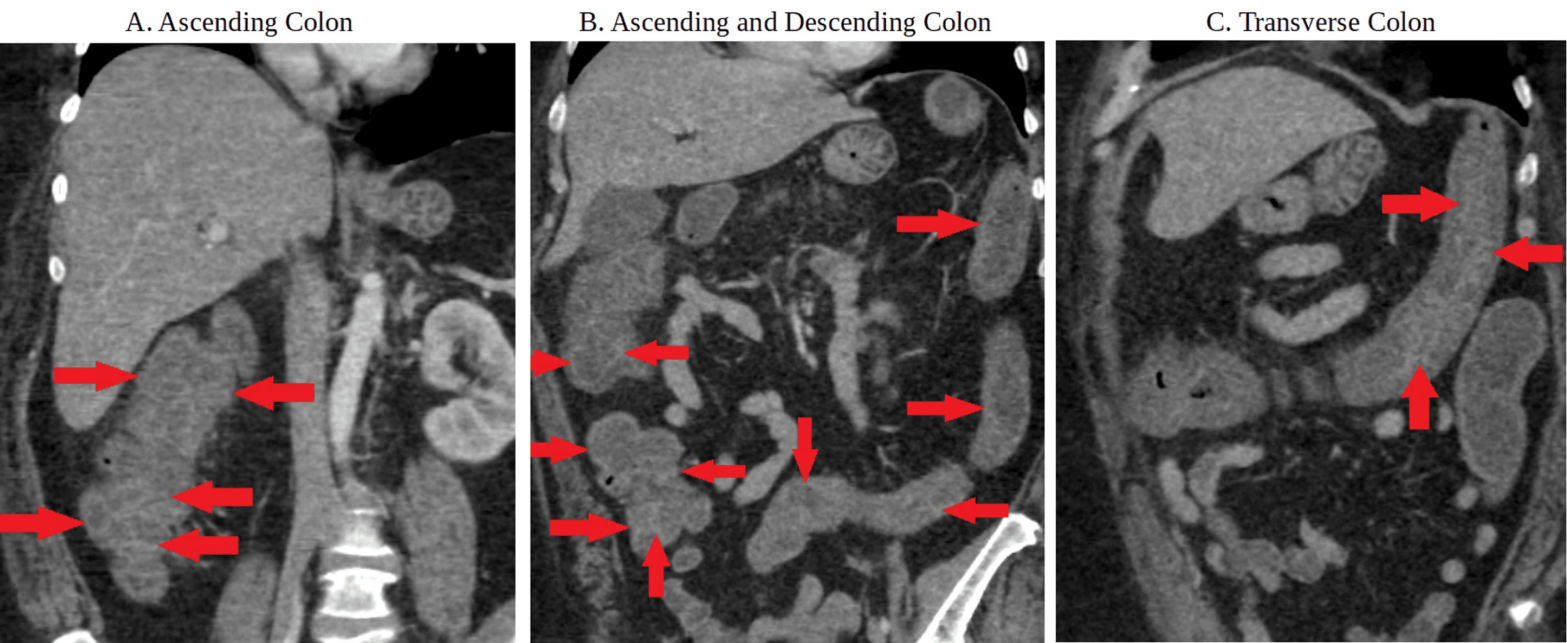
Computed tomography abdomen and pelvis with contrast There is circumferential, moderate, wall thickening of the ascending, transverse, and descending colon consistent with colitis with the red arrows delineating contrast being trapped between edematous haustral folds also known as the “accordion” sign.
